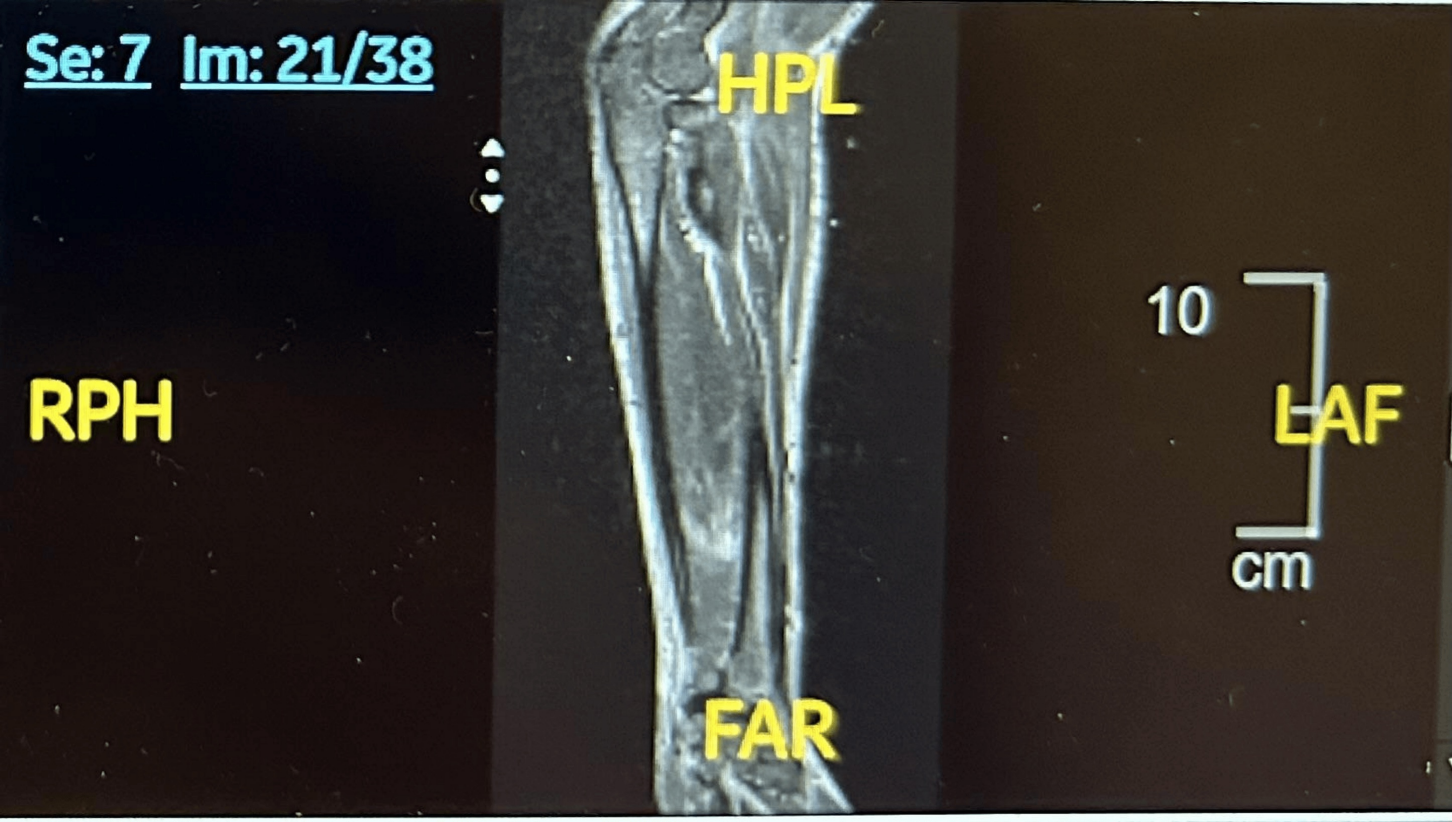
Coronal MRI of the right forearm demonstrates hyperintense signal along the deep fascial planes, consistent with diffuse fasciitis, particularly in the flexor compartment.
